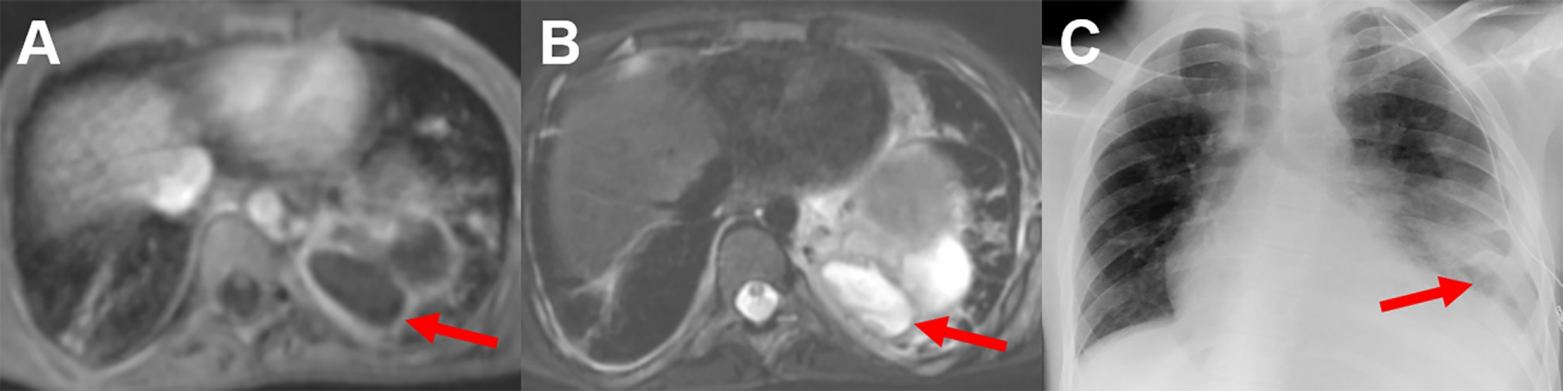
Contrast-enhanced T1 weighted sequence in comparison with T2 weighted sequence of a 7-year-old child. Both MRI sequences clearly delineate an abscess formation in the left lower lobe. On T1-weighting imaging (A) the abscess is characterized by the missing central contrast uptake and peripheral rim enhancement (red arrow). On T2-weighting (b), the necrotic center of the abscess has a high signal intensity, whereas the thick capsule shows a lower intensity (red arrow). The corresponding CXR (c) shows a consolidation in the left lower lung field as well as the abscess (red arrow). The example shows that the extension of abscess formations might be underestimated in CXR.
